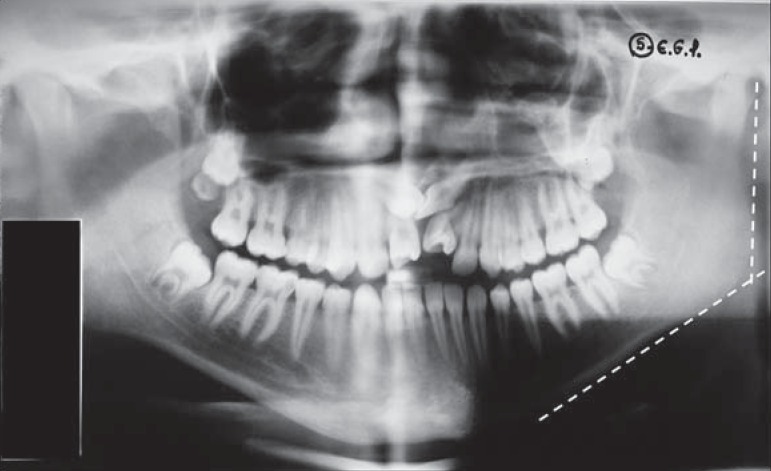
Panoramic radiography with the mandibular angle lines
